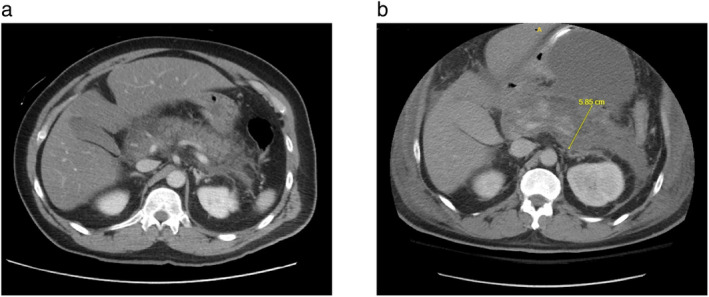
(a) Computed tomography of the abdomen and pelvis with intravenous contrast consistent with acute interstitial pancreatitis with severe hepatic steatosis and hepatomegaly. (b) Computed tomography of the abdomen and pelvis with intravenous contrast consistent with necrotizing pancreatitis with peripancreatic fluid collection, partially loculated fluid within the left anterior pararenal space, increased acute necrotic collection exerting increased mass effect on the adjacent stomach, increasing loculation and peripheral enhancement of the collection, and interval increase in the amount of intra‐abdominal ascites, specifically in the perihepatic space.
